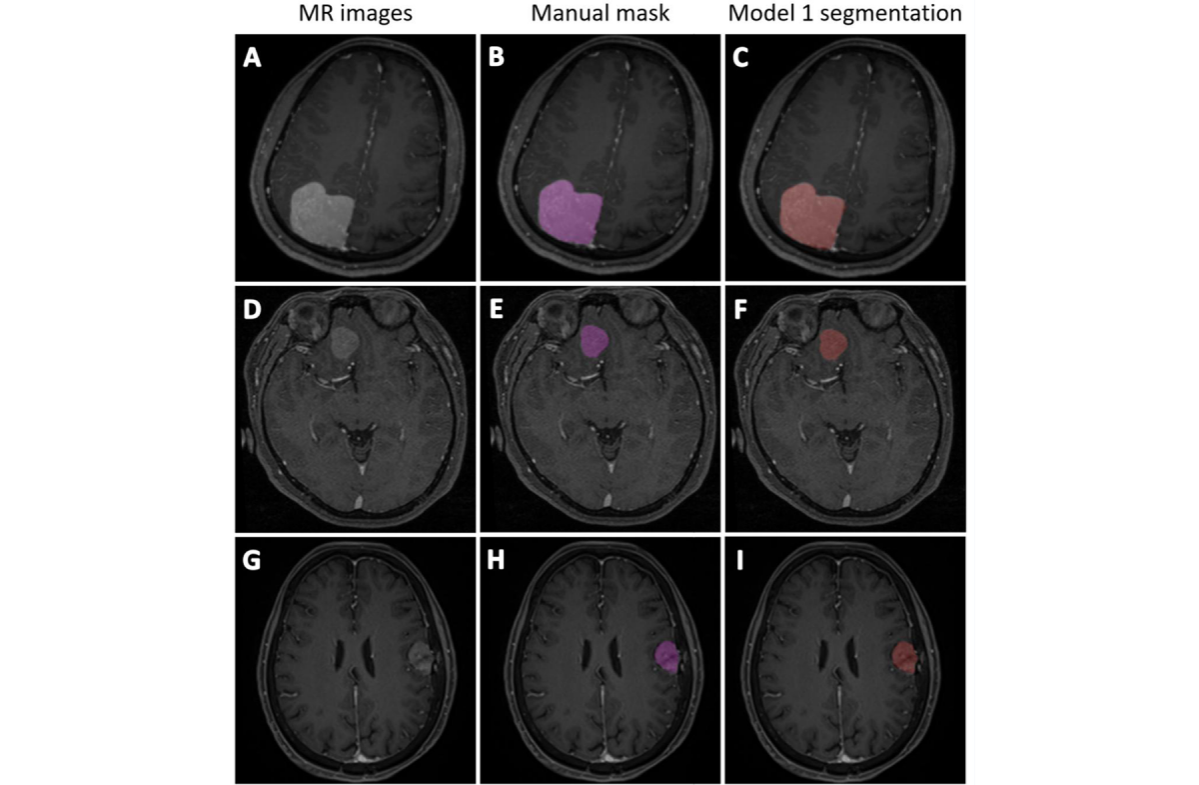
The examples of magnetic resonance imaging from 4 databases. (A) Center A (magnetization-prepared rapid gradient echos [MPR-AGEs]); (B-C) center B (MPR-AGEs); (D-G) center C (fat-suppressed fast or turbo spin echos [FSEs/TSEs]); (H) center D (FSEs/TSEs). Tumor boundary is more clear in MPR-AGEs as they present high spatial resolution (red arrow). Moreover, the cerebral cortex is rather obvious in MPR-AGEs but not in FSEs/TSEs, as FSEs/TSEs are fat suppressed (yellow arrow). MR: magnetic resonance.
